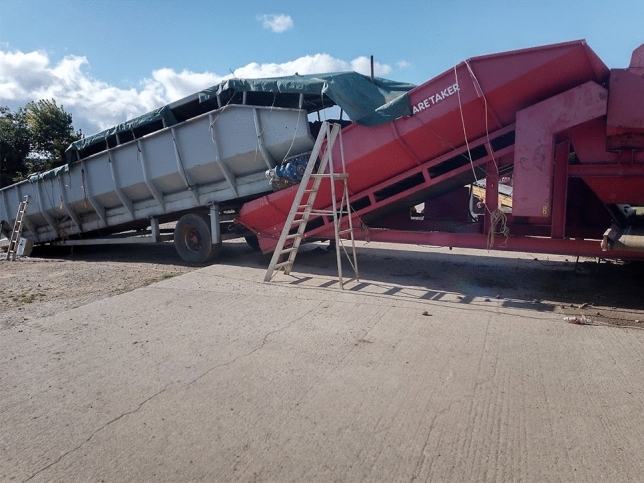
Farmer invention: feed potato grader to hold potatoes. Farmer description:“ The grey hopper holds 14tons of potatoes and was previously part of a carrot line in a supermarket packhouse. We built the chassis and fitted the axle that its now on to make it mobile and suitable to fit into our system. It starts and stops automatically via an ultrasonic sensor to feed the grader on demand, and its capacity has saved us a tractor, trailer and operator all season, worth today about £400/day. It cost £11,500 to build including the modifications
